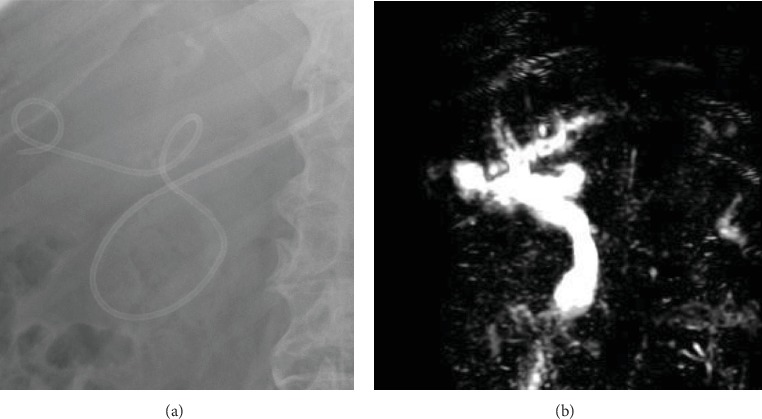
Images of Case 2. (a) X-ray image showing successful placement of a curved nasobiliary duct into the gallbladder cavity. (b) Magnetic resonance cholangiopancreatography (MRCP) image showing that gallbladder volume was reduced postoperatively, with a significant decrease in compression of the common bile duct.
